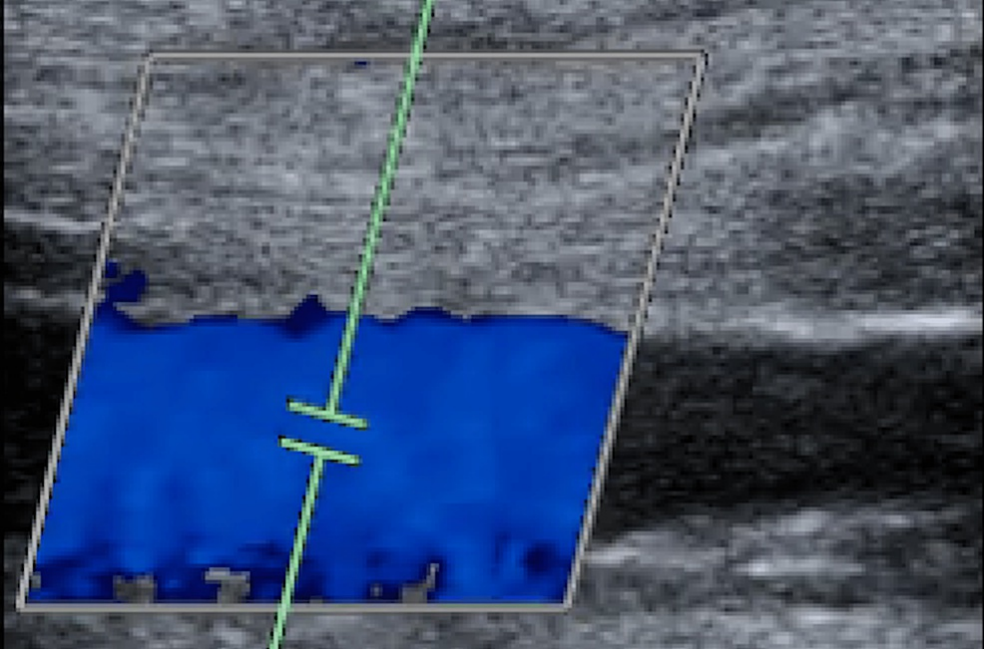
Venous doppler of the left lower extremity.
